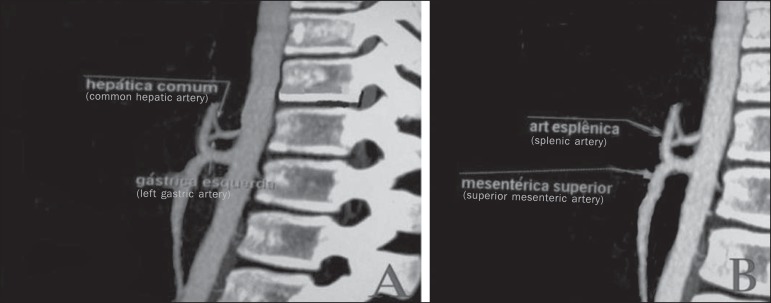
Contrast-enhanced sagittal computed tomography. A: The image
